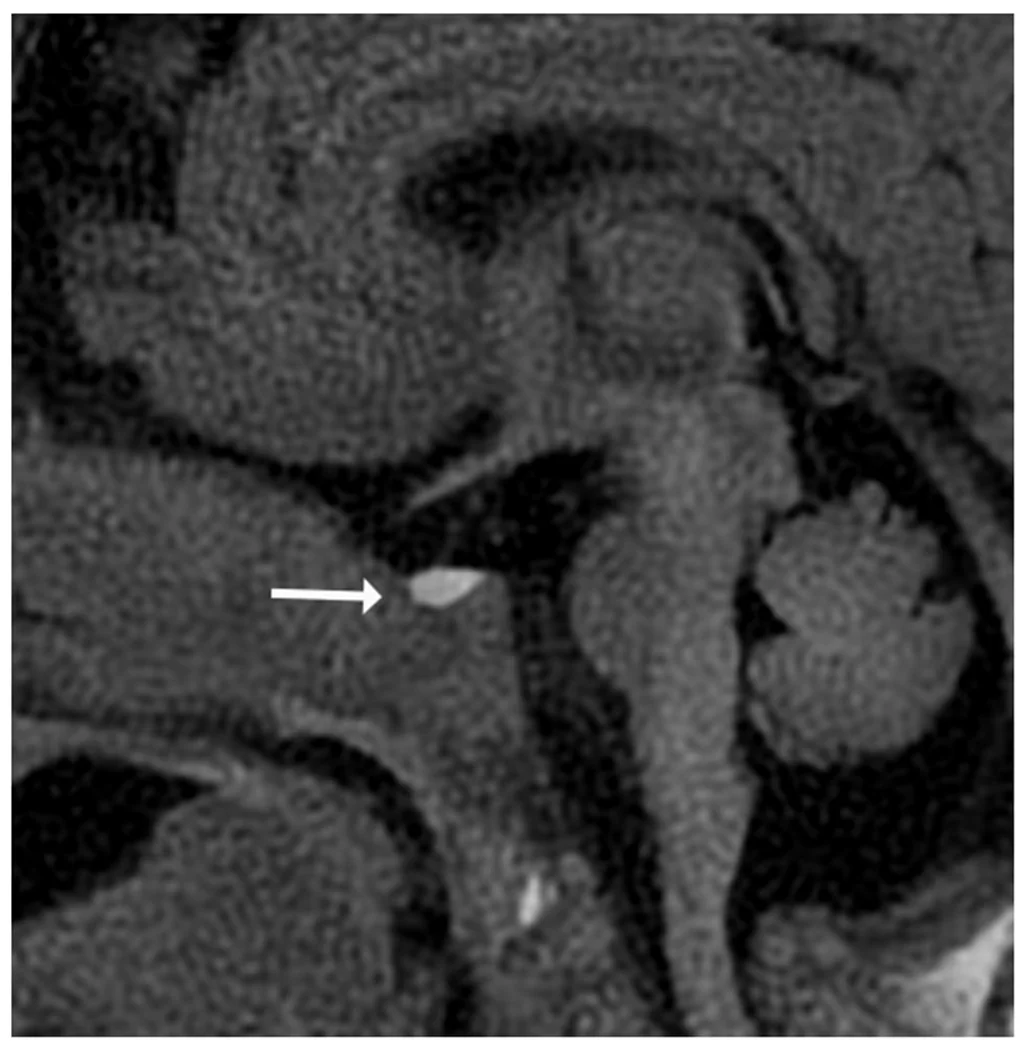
Normal appearance of adenohypophysis in a 29-day-old male infant with seizures (no endocrinopathy). Sagittal T1-weighted brain magnetic resonance imaging focused on the pituitary gland demonstrates a uniformly hyperintense adenohypophysis (*arrow*)
